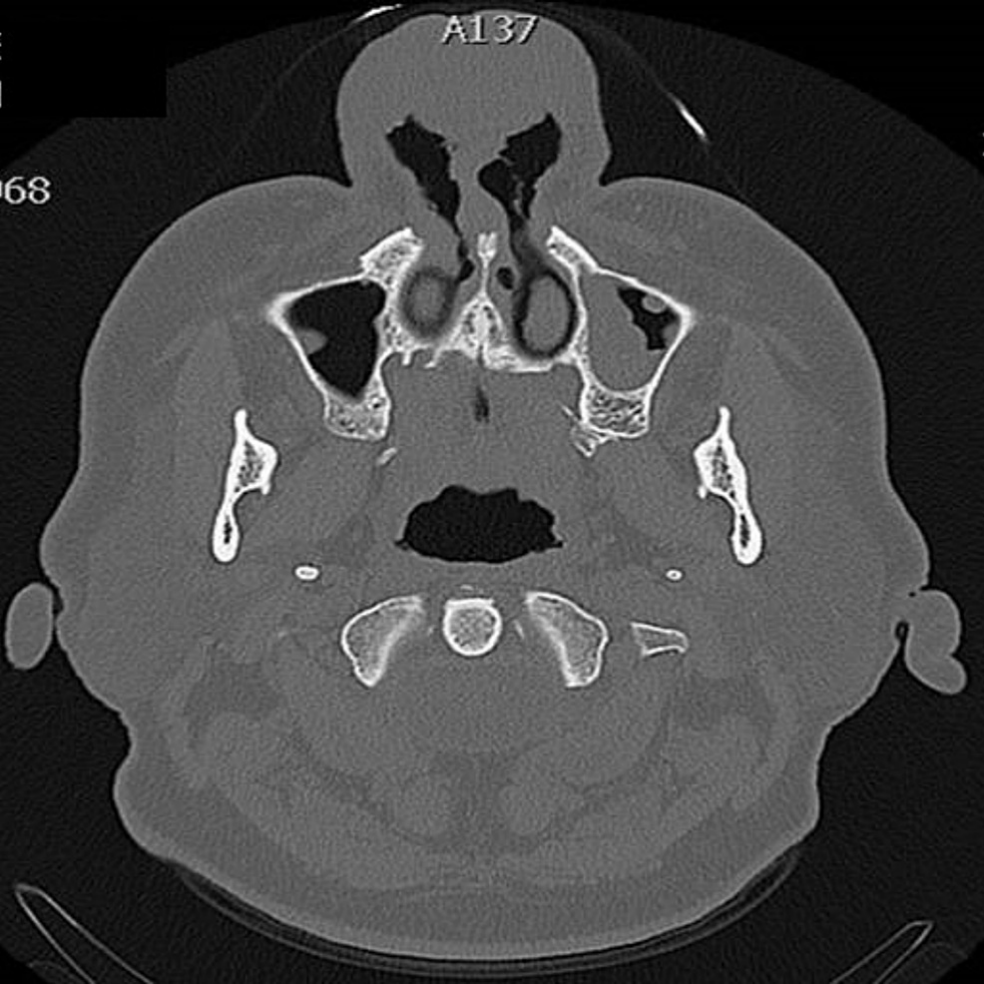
Axial computer tomography (CT) shows thickening of the skin, the subcutaneous fat and the cartilages of the nose.
